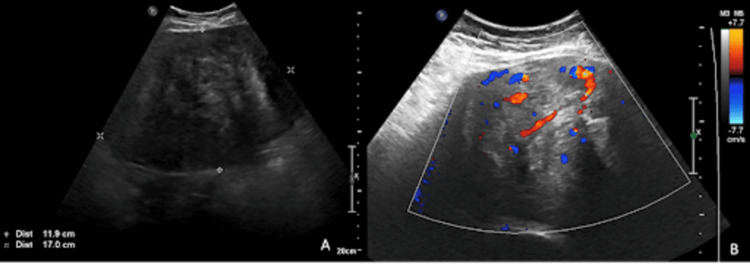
B-mode transabdominal ultrasound image (A) and color Doppler image (B) demonstrating a large well-demarcated heterogeneously hypoechoic abdominopelvic mass lesion with internal vascularity.
